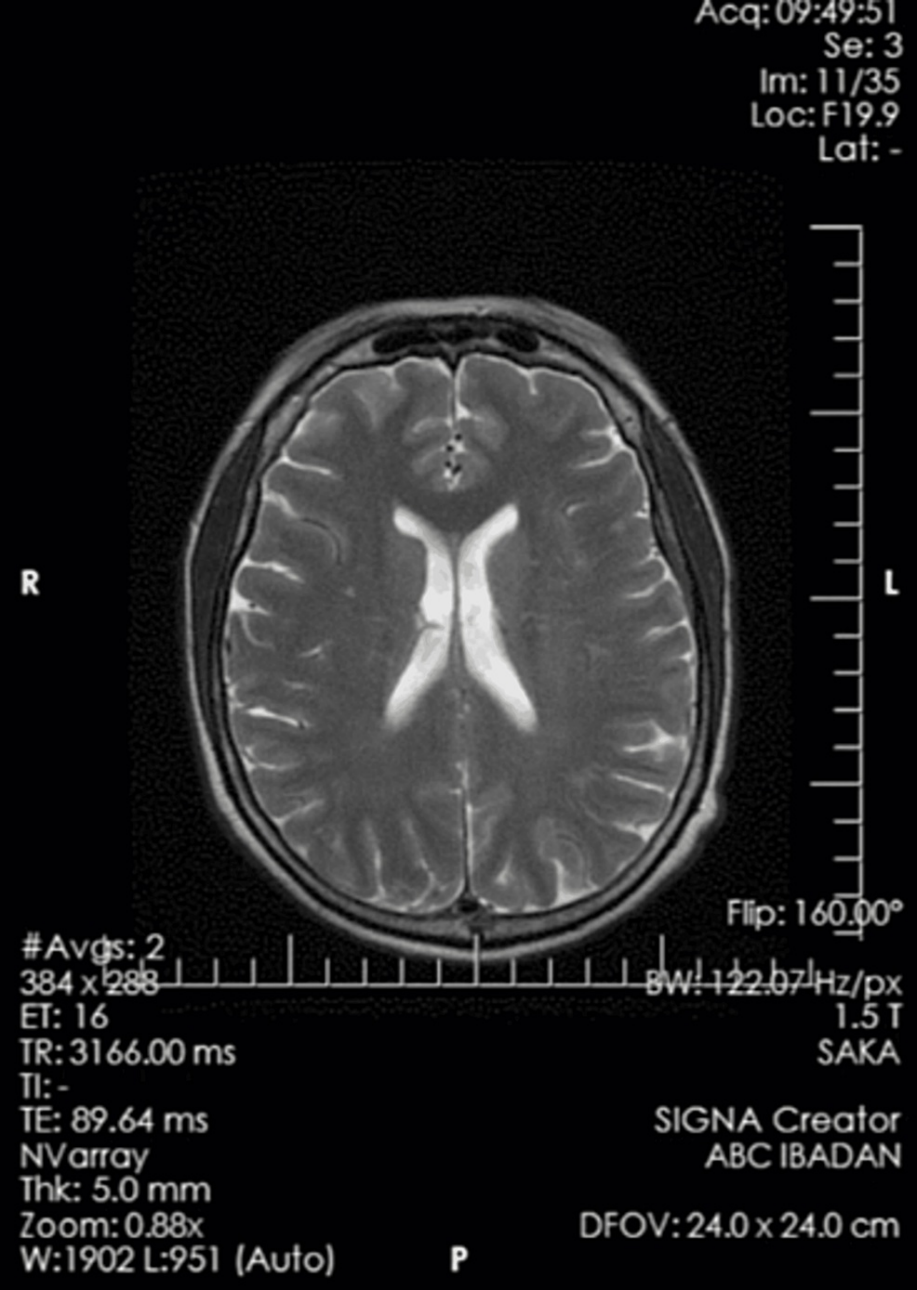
An axial view of a T2WI brain MRI scan with normal findings. T2WI: T2-weighted images; MRI: Magnetic resonance imaging.
